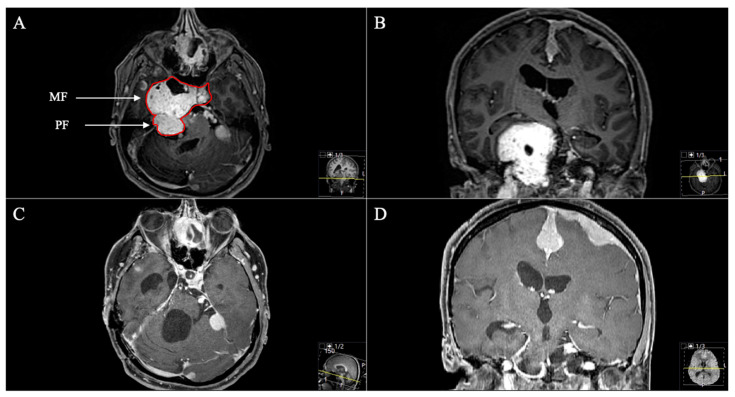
A 39-year-old male patient with neurofibromatosis type 2 presented with progressive vertigo, intermittent right facial pain and trigeminal hypesthesia (V1). (A) Preoperative axial and (B) coronal T1-weighted gadolinium-enhanced MRI, showing an impressive space-occupying cystic trigeminal schwannoma, involving the middle (MF) and posterior fossa (PF) through Meckel’s cave (Samii Type C). (C) Postoperative axial and (D) coronal MRI control, indicating complete resection via a two-stage technique; a modified Kawase and a retrosigmoid approach were performed. Postoperatively, no new deficits occurred and the patient recovered from the facial pain.
